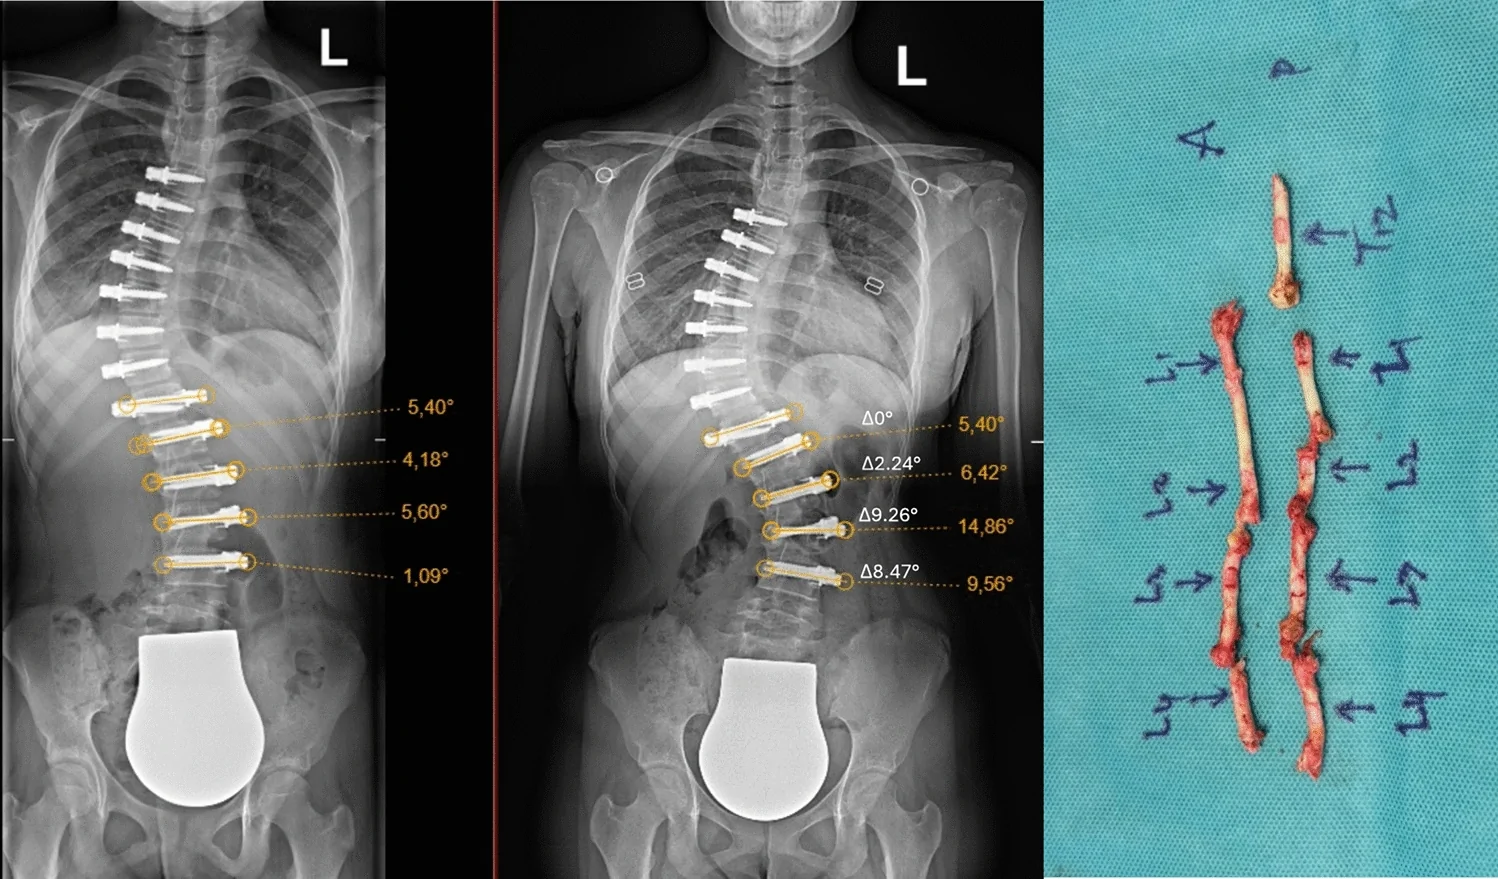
Example case of a 11 + 7-year-old girl who has had double VBT at Risser 0 and Sanders Stage 3. 1st erect and 27 months post-op radiographs suspected tether breakages at L2/3 and L3/4. Intra-op explantation additionally identified breakages of the posterior tether at T12/L1 and L1/2
